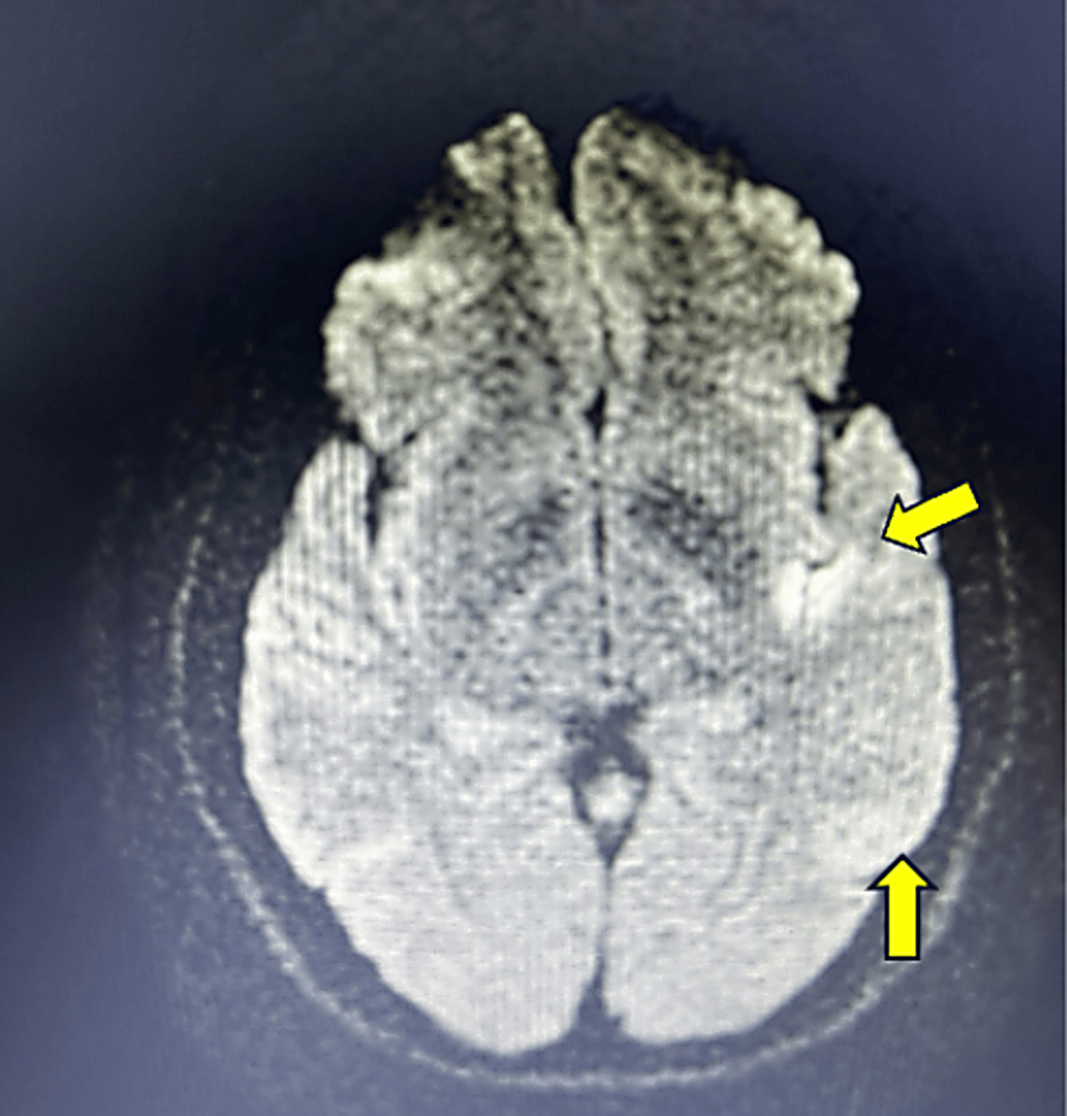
A T2-weighted MRI revealed a hyperintense signal consistent with acute infarcts in the left temporal and parietal regions
